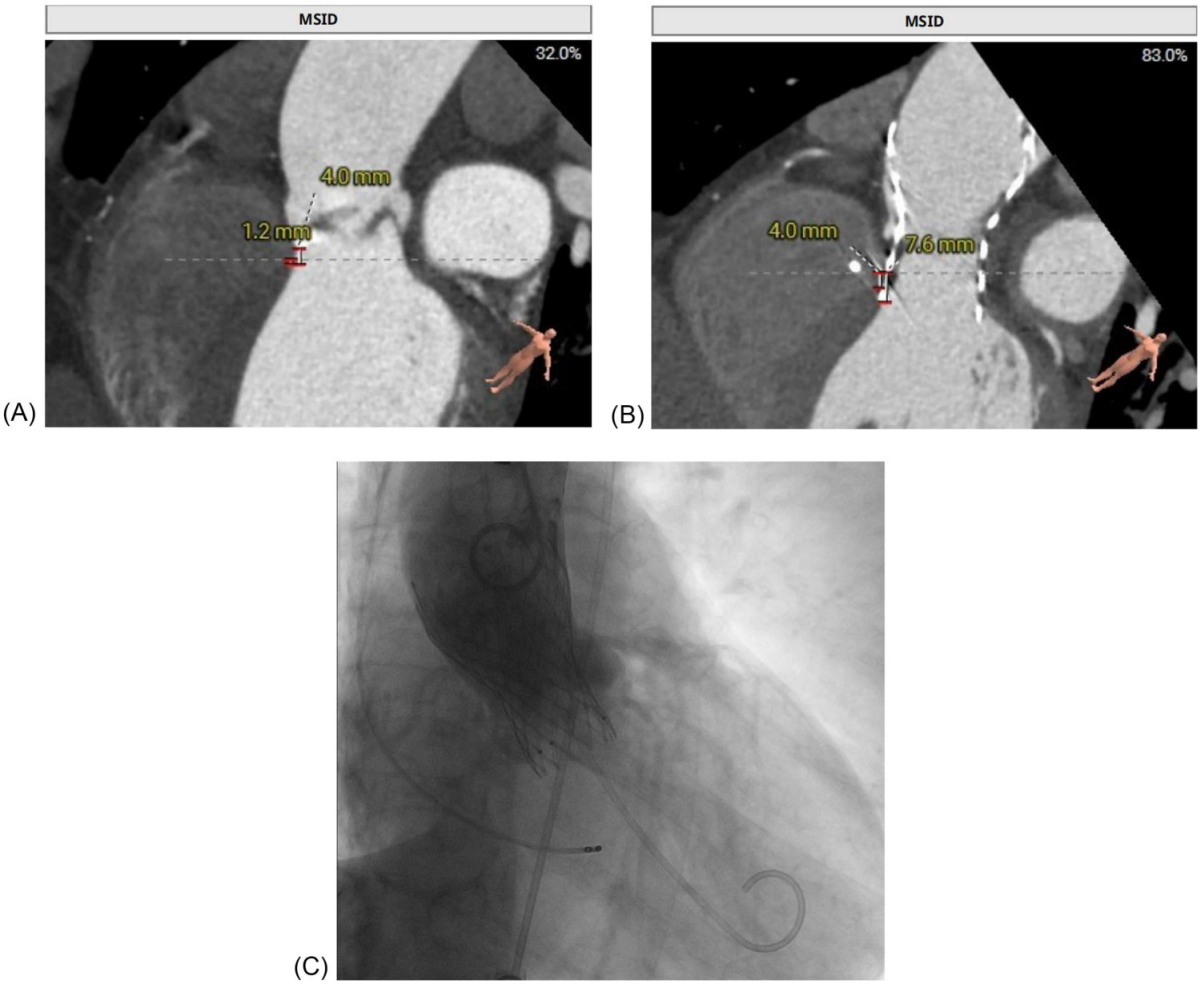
Multimodal imaging of a patient with delayed atrioventricular block after TAVR replacement. (A) Preprocedural CT demonstrated a short membranous septum (4.0 mm) and a membranous septum–annulus distance of 1.2 mm, consistent with a high conduction risk. (B) Post-event CT showed further valve expansion, with a frame depth of 7.6 mm at the membranous septum plane and 9.8 mm at the non-coronary cusp, consistent with progressive compression of the conduction system. (C) Fluoroscopic image during transfemoral TAVR, confirming deployment of the self-expanding Qiming L26 prosthesis at a depth of approximately 5 mm.
